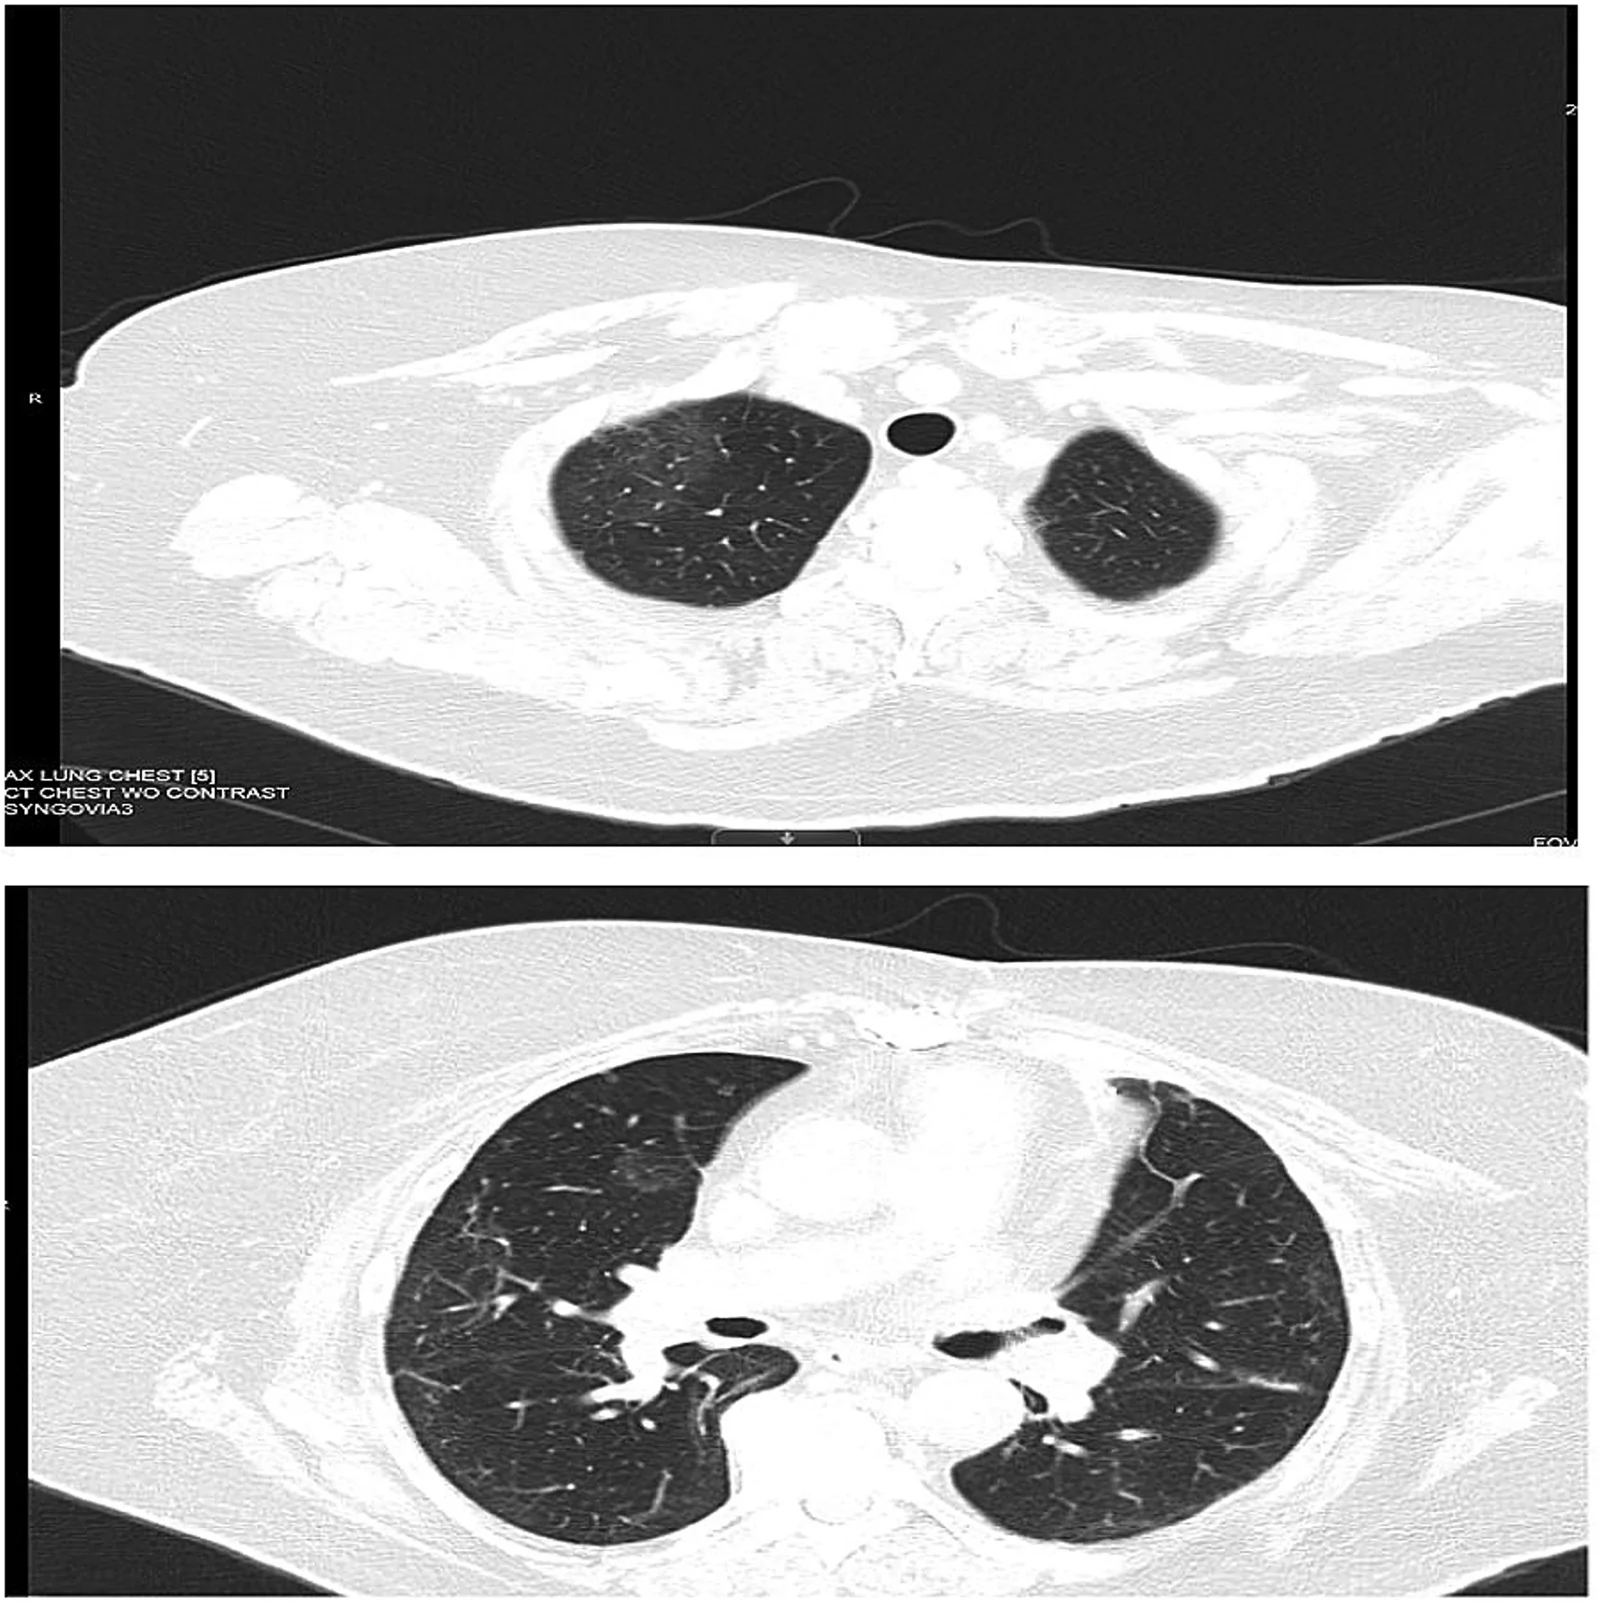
CT without contrast, 6 weeks after discontinuing Daptomycin, showing resolution of prior changes.
